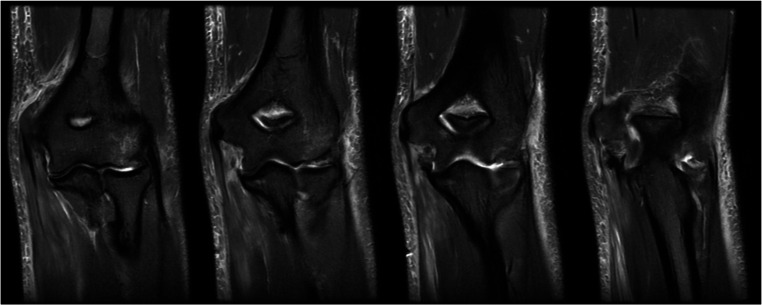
Coronal proton density weighted MRI series demonstrating a mid-substance tear of the ulnar collateral ligament
